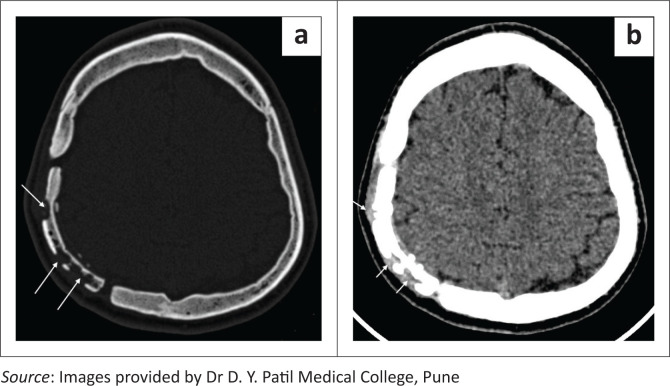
Bone flap infection. Axial bone window CT image in a patient with a right parietal craniotomy demonstrates a mildly sclerosed bone flap with multiple well defined lytic areas (a, long white arrows) and a small adjacent soft tissue component (b, short white arrows).
